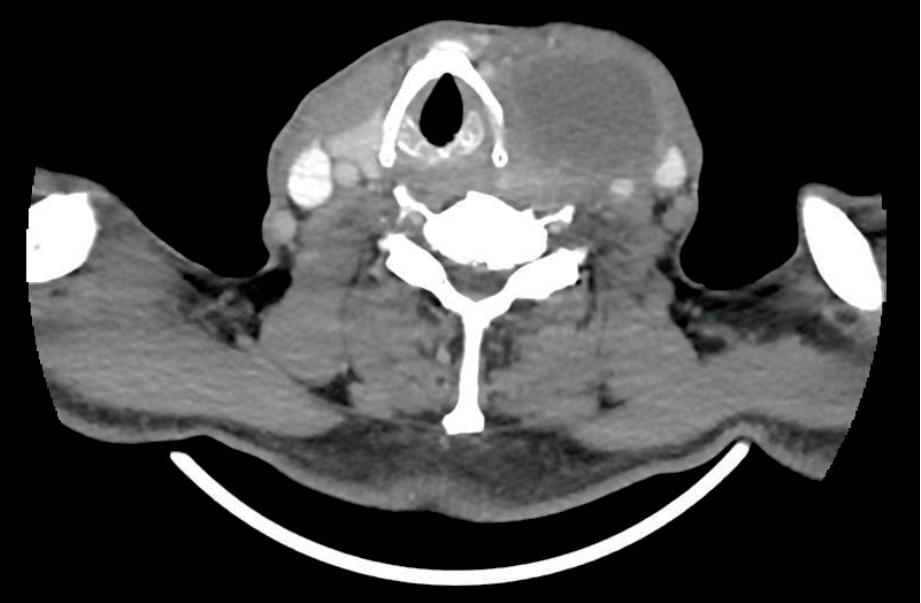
CT scan (axial) showing a large hypoechoic nodule with ill-defined nodular margins. This is suggestive of a solid-cystic nodule in the left thyroid.
